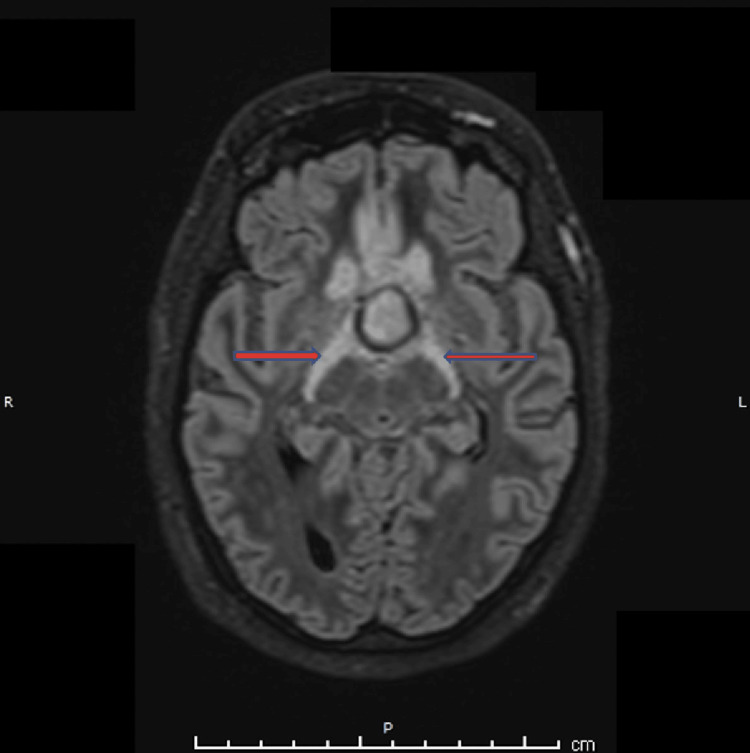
Axial 3D SPACE FLAIR image demonstrating a sellar–suprasellar pituitary macroadenoma with symmetrical hyperintensity along the bilateral optic tracts (arrows), consistent with optic tract edema (“moustache sign”). FLAIR: Fluid-attenuated inversion recovery; SPACE: Sampling Perfection with Application optimized Contrasts using different flip angle Evolution
